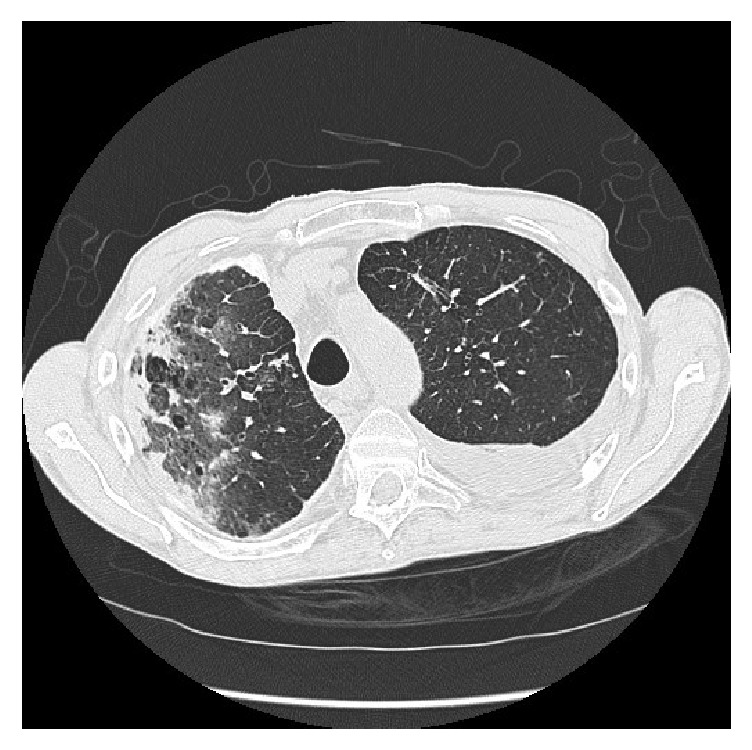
CT scan of chest, showing right airspace shadowing, bronchiectasis and diffuse pleural thickening.
